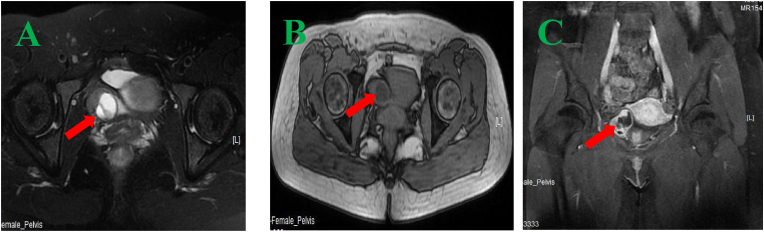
MRI image (A–C): A cystic-solid mass (31.9 mm × 24.5 mm × 37.4 mm) located in the right side of the pelvis (between the right ovary, uterus, and bladder), with enhancement of the solid part observed on the enhanced scan.
